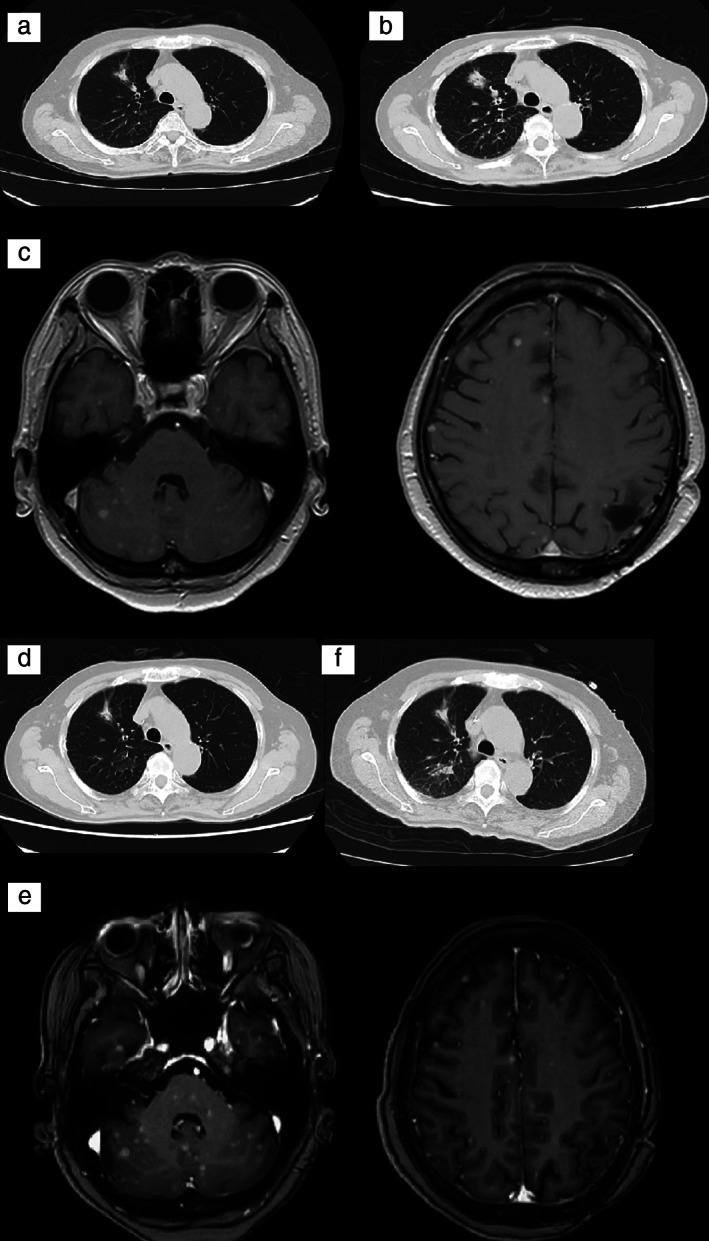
Computed tomography (CT) and magnetic resonance imaging (MRI) were undertaken during the clinical course. (a) After two courses of chemotherapy, CT revealed that the lung tumor had shrunk. (b) CT confirmed an increase in the lung tumor after five months. (c) Recurrence of multiple brain metastases was evident on MRI scan. (d) After one month of afatinib treatment, CT confirmed that the primary lung tumor had shrunk. (e) After one month of afatinib treatment, MRI revealed that the brain metastases had worsened. (f) After two months of afatinib treatment, CT showed an increase in the primary lung tumor
